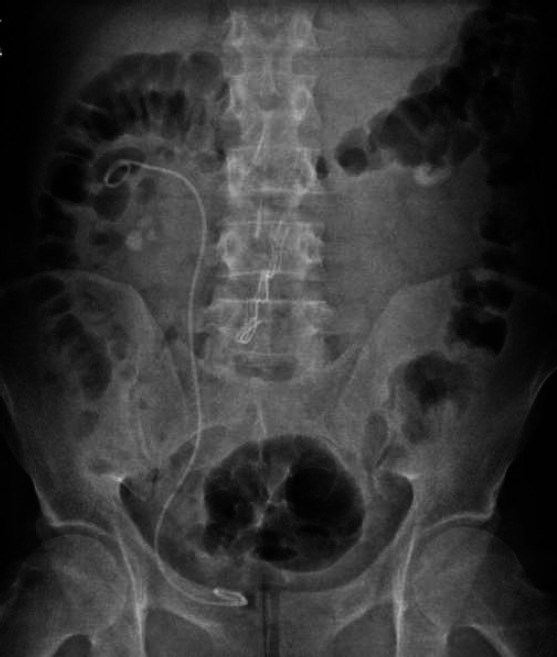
KUB radiography taken on the same day after the operation.
